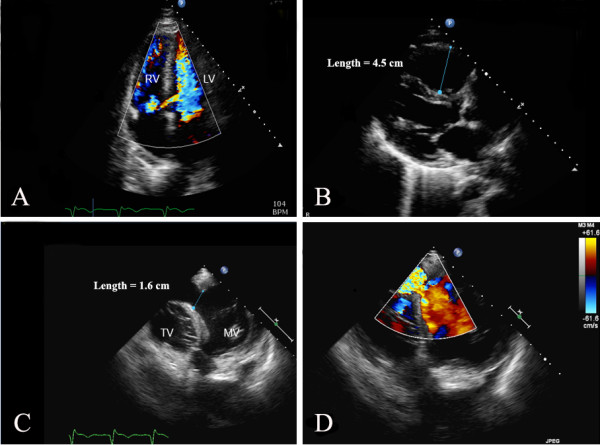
Echocardiogram.A) Transthoracic echocardiogram in the apical 4 chamber view showing a ventricular septal rupture in the inferobasal myocardial septum with left-to-right shunt by color Doppler mapping. B) Transthoracic echocardiogram in the parasternal long axis view showing significant right ventricular enlargement (proximal right ventricular outflow tract in this image) secondary to left-to-right shunt and volume overload. C) Transesophageal echocardiogram from a modified transgastric view at 0° demonstrates a 1.6 cm defect in the inferobasal septum at the level of the mitral valve (MV) and tricuspid valve (TV). D) High velocity turbulent flow of left-to-right shunt by color Doppler across the ventricular septal rupture noted in C.
